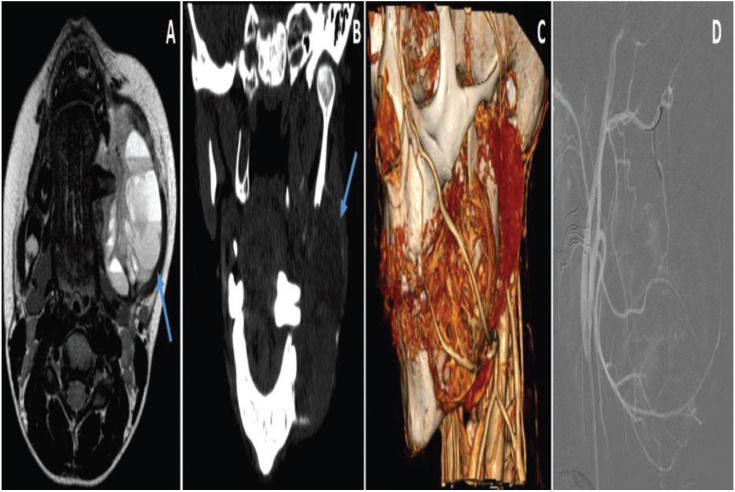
A: Axial T2 MRI (Magnetic Resonance Imaging) shows fluid-fluid levels characteristic of ABC (Arrow). B: CT (Computerized tomography) coronal view, shows a corticated (Arrow) ballon-like expansile lesion in the left posterior mandible with tooth desplazement. C: Lateral view of CT Angiography volume rendering. D: Angiography showing embolization procedure.
